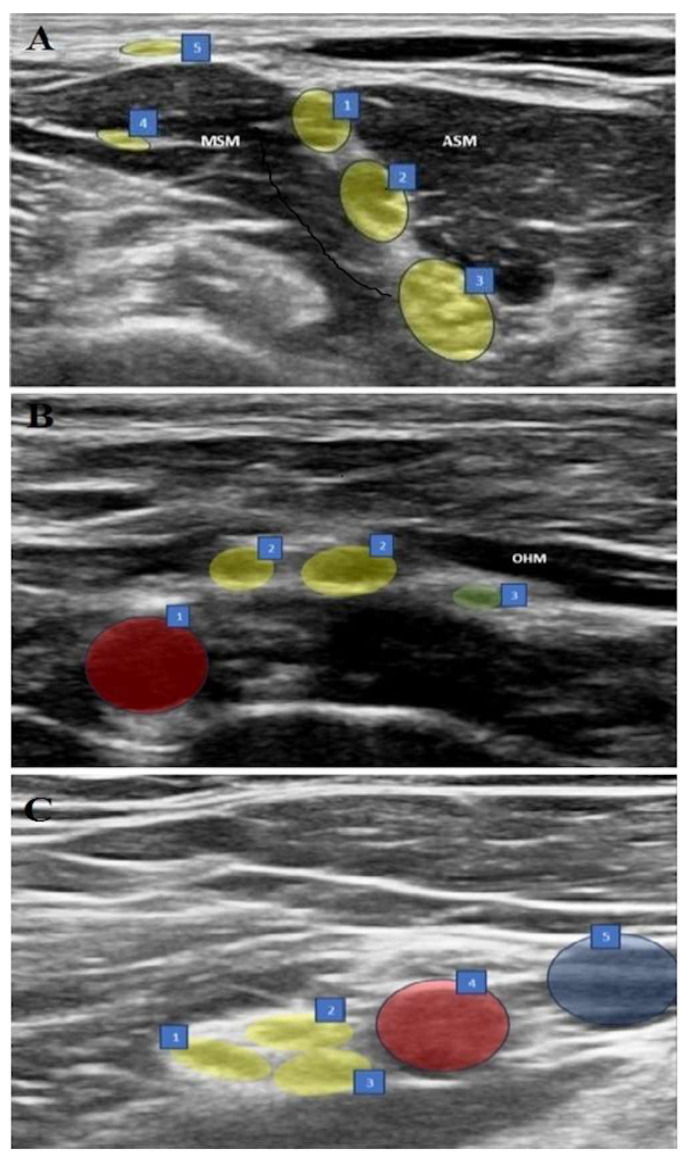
(A–C) Sonographic views of three blocks. (A) ISB: 1. C5 nerve root, 2. C6 nerve root, 3. C7 nerve root, 4. Long thoracic nerve, 5. Supraclavicular nerves, ASM: Anterior scalene muscle, MSM: Middle scalene muscle. (B) ASB: 1. Subclavian artery, 2. Upper trunk, 3. Suprascapular nerve, OHM: omohyoid muscle. (C) CCB: 1. Posterior cord, 2. Lateral cord, 3. Medial cord, 4. Axillary artery, 5. Axillary vein.
